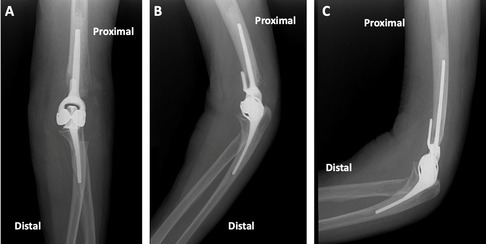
Final postoperative radiographs. Anteroposterior (A), oblique (B), and lateral (C) radiographs showing a left elbow total elbow arthroplasty semiconstrained components in proper positioning.
